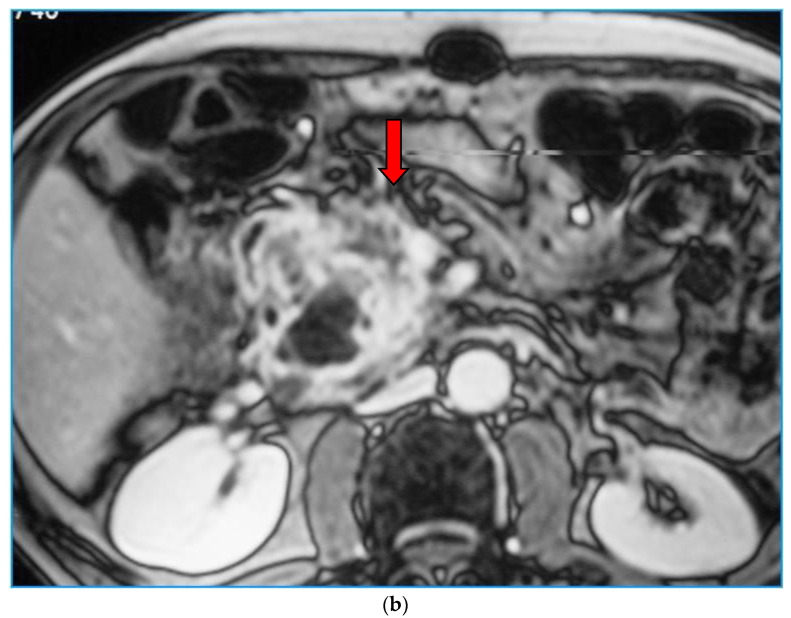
Contrast-enhanced CT image (a) and T1-w MR image post iv contrast administration (b), show an IPMN with invasive carcinoma (arrow) in the pancreatic head infiltrating the superior mesenteric vein.
